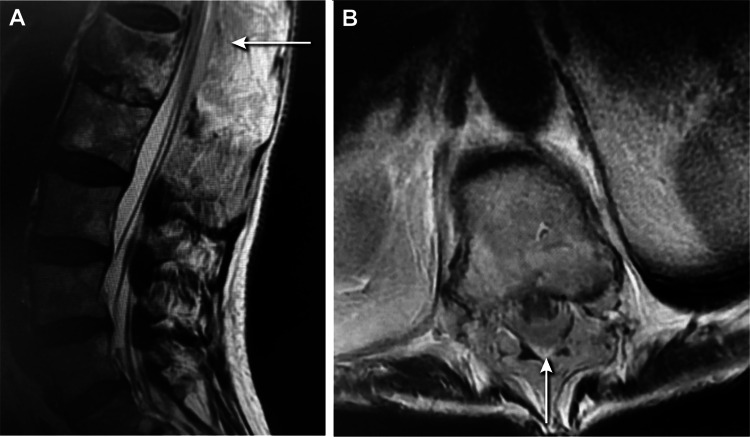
Preoperative MRI of the lumbar spine in case 1. Sagittal (A) and axial (B) preoperative imaging showing spinal epidural hematoma and spinal subdural hematoma (white arrows). Used with permission from Barrow Neurological Institute, Phoenix, Arizona.
